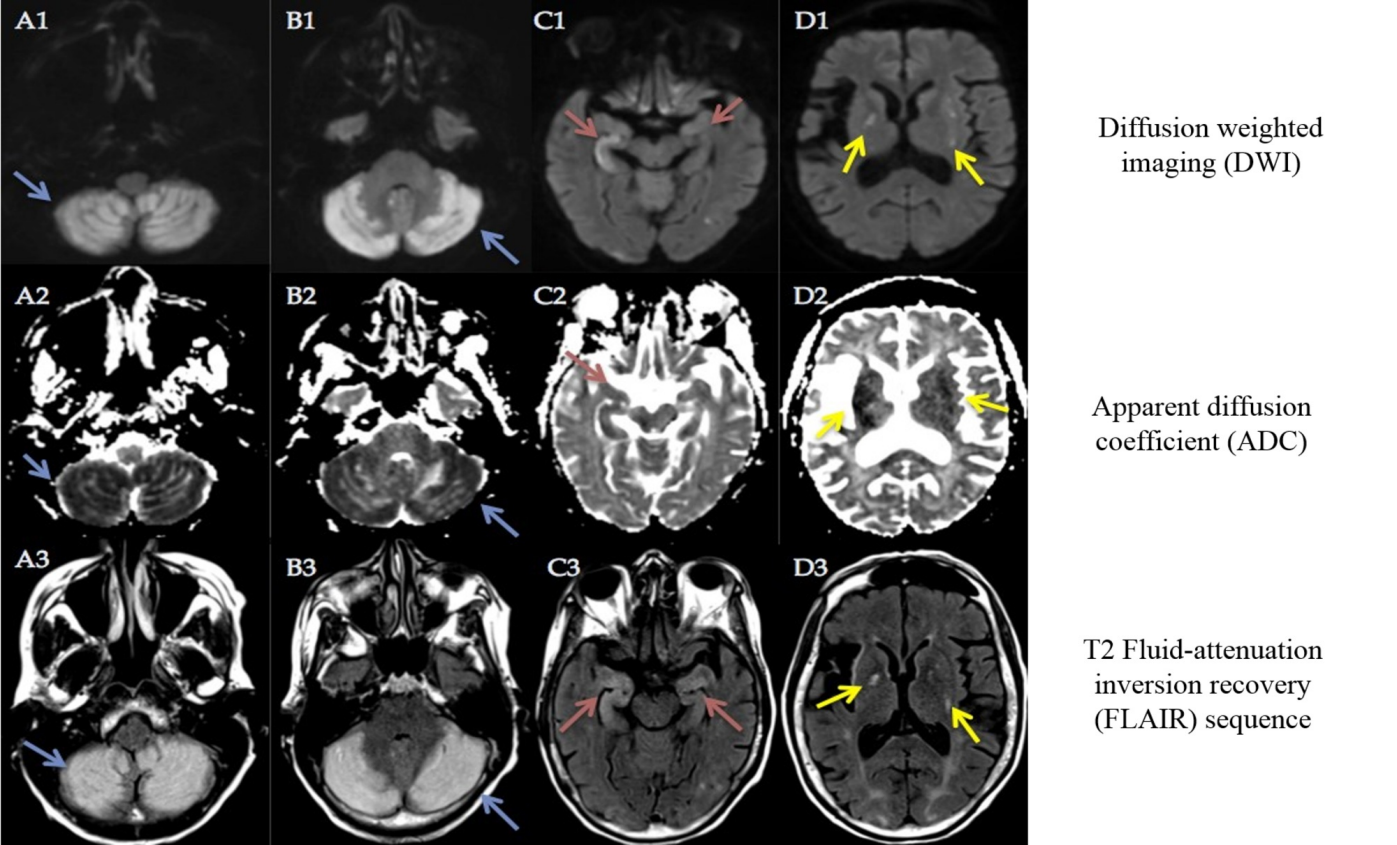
Magnetic resonance imaging (MRI) of the brain Magnetic resonance imaging of the brain DWI, ADC and T2 FLAIR sequences of the corresponding axial sections at cerebellar hemispheres (A1, A2, A3, B1, B2, B3), hippocampi (C1, C2, C3), and basal ganglia (D1, D2, D3) showing diffusion restriction and hyperintensity in the bilateral posterior inferior cerebellar artery (PICA) (blue arrows), hippocampi (red arrows), and basal ganglia regions (yellow arrows) respectively.
